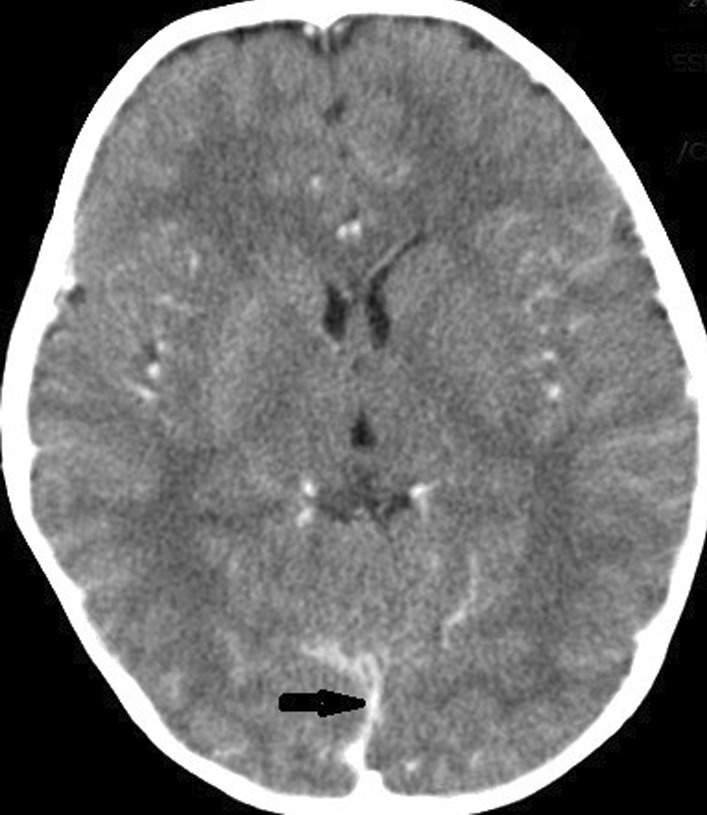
A computed tomography scan of brain shows generalized meningeal enhancement (The arrow points towards meningeal enhancement)
